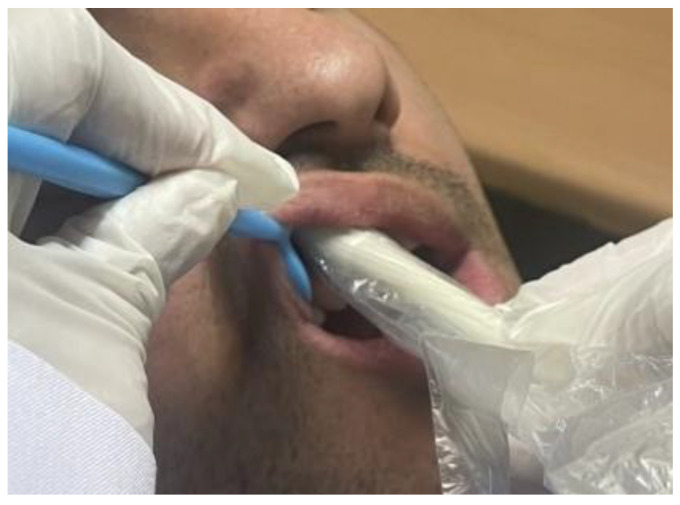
Measurement of gingival thickness using an intraoral ultrasonographic transducer.
